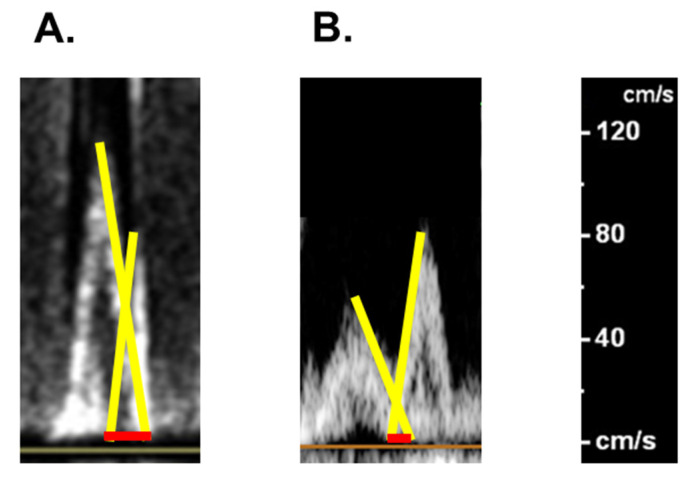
Trans-mitral left ventricular inflows obtained by Doppler echocardiography on admission (A) and following the up-titration of ivabradine (B). The overlap between the two waves are presented as red bars.
